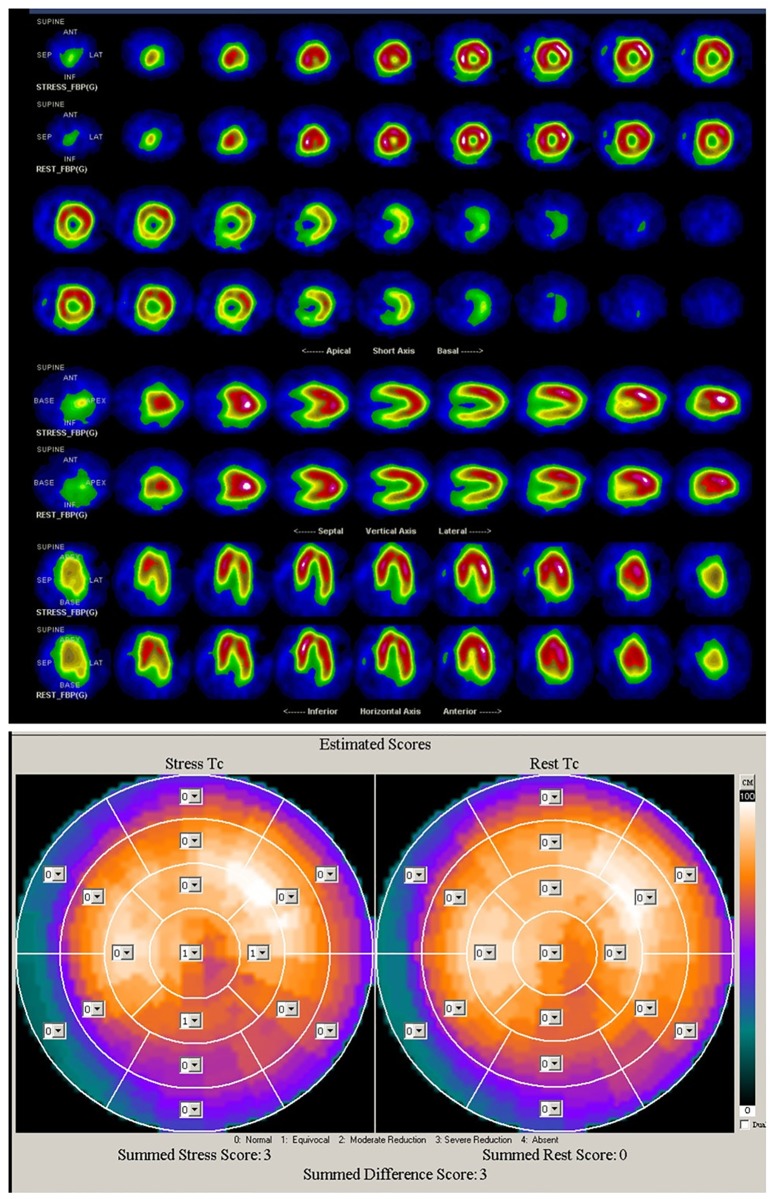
SPECT MPI results of GB0527. The rest and stress SPECT ischemia score of GB0527 are 0 and 3, respectively.
